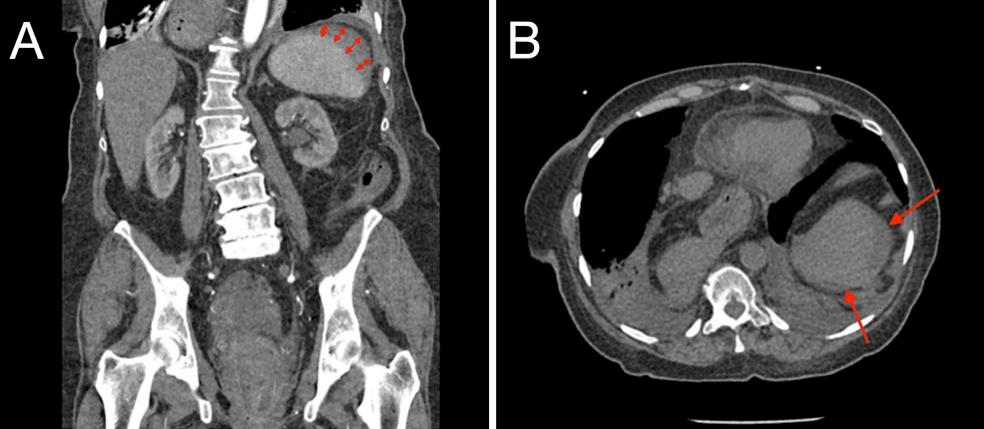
Abdominal CT scans (A, coronal and B, axial) The CT scans displayed in images A and B reveal a splenic hematoma. In Figure 1A, the double-headed arrows indicate the extent of the hematoma as seen in the coronal plane. Figure 1B uses two arrows to delineate the margins of the hematoma in the axial plane. The radiological report concluded a grade 2 splenic laceration with a peri-splenic hematoma measuring 8 cm.
